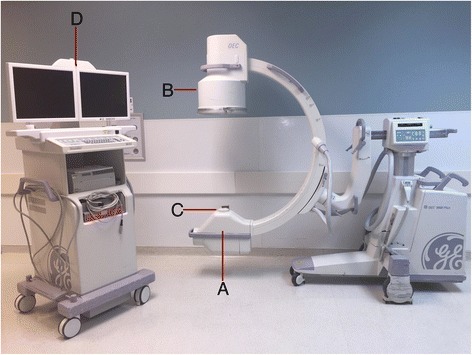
Basic c-arm unit. a. X-ray Tube; b. Image Intensifier; c. Collimator; d. Display Monitor
